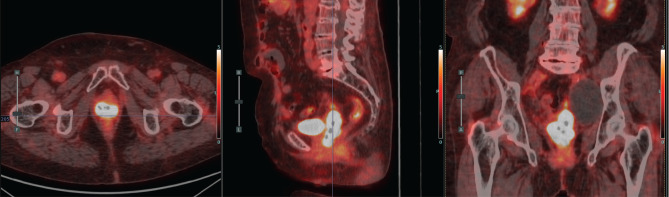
Case 2. PET-CT scan prior to BT. Axial, sagittal and coronal section. A hypermetabolic tumour mass in the vaginal vault and a left pelvic lymphocele were observed.
